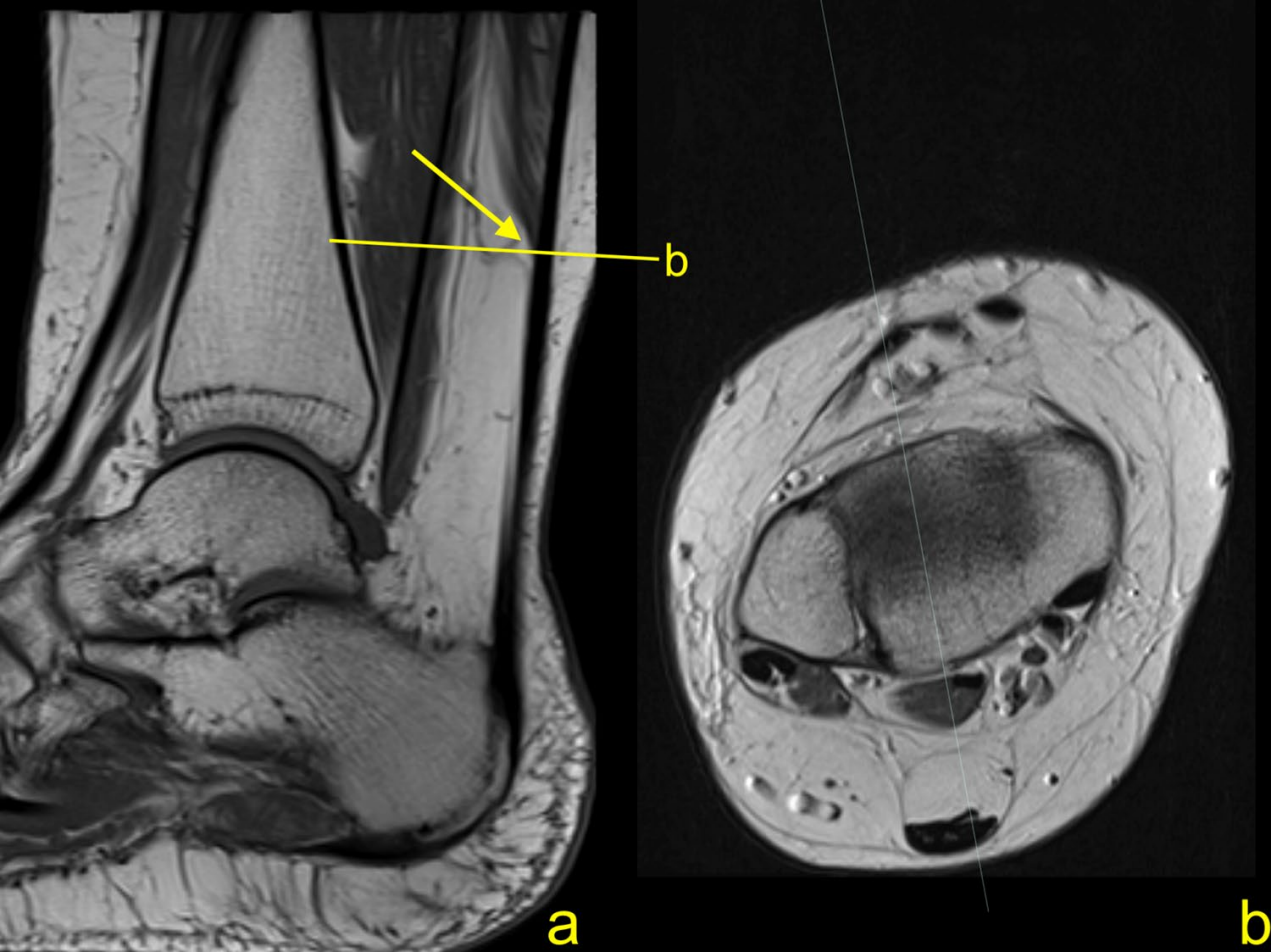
A 39-year-old male with clinical suspicion of the posterior impingement, normal Achilles tendon and a long free tendinous part measuring 71 mm. MRI (a T1−w TSE (turbo spin echo), b PD−w) showed normal Achilles tendon. The lowest level where the soleus muscle fibers can be identified is marked with an arrow (a)
